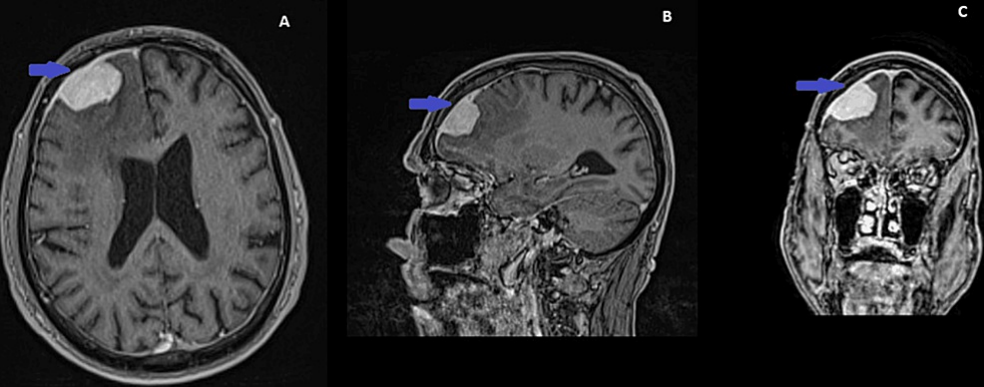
(A-C) MRI of the brain with contrast material (gadolinium) in T1 projections of axial, sagittal, and coronal sections shows a well-defined and intensely saturated hyperintense, homogeneous tumor mass located in the right frontal lobe, captured in a wide base for the convexity of the brain shell with dimensions: 2.96/1.83 cm; 2.59/2.01 cm; 3.21/2.15 cm.
